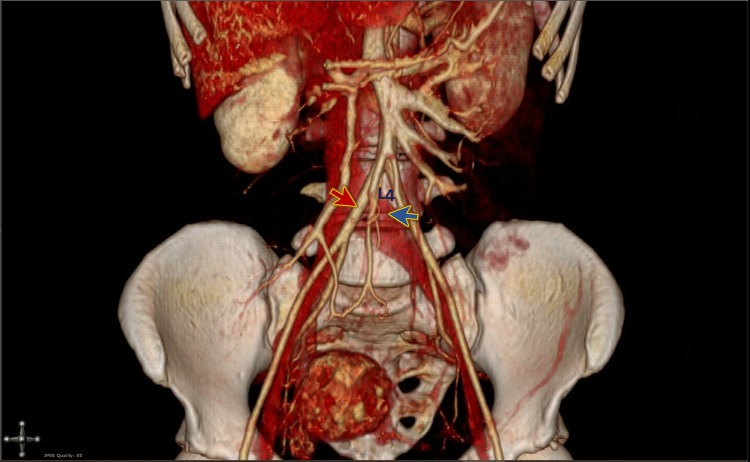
3D reconstruction of the CT venogram shows compression of the left iliac vein (blue arrow) by the right iliac artery (red arrow) at the level of the 4th lumbar vertebra (L4).
